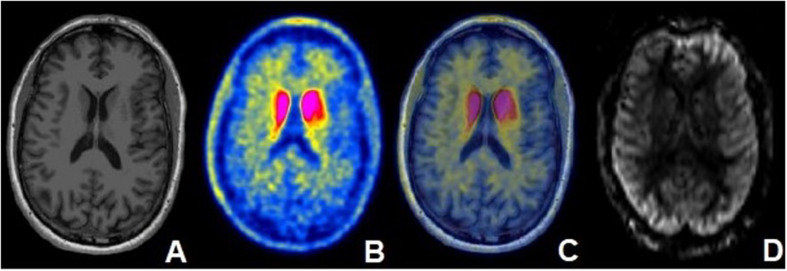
Fifty five year old male with PD diagnosed 5 years ago with moderate disease based on MDS-UPDRS part 3 (motor) “off” score and associated mild cognitive impairment. (A) Axial T1-weighted MRI demonstrating no structural abnormalities. (B) Axial [11C]-PE2I PET image and (C) fused axial [11C]-PE2I PET/MR image demonstrating increased PE2I avidity in the bilateral caudate nuclei. (D) rCBF map obtained in the same patient at the same level.
